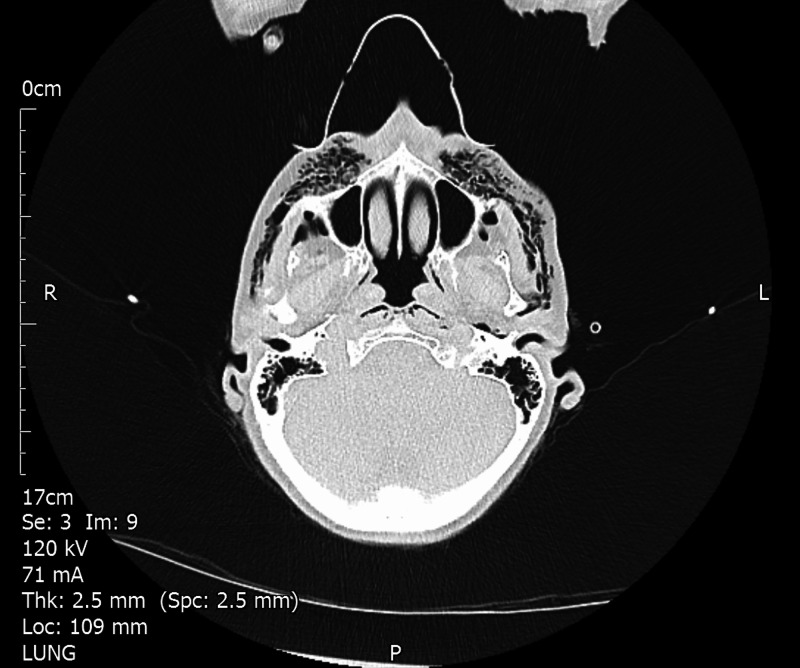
CT showing pockets of subcutaneous overlying the maxilla bilaterally.
